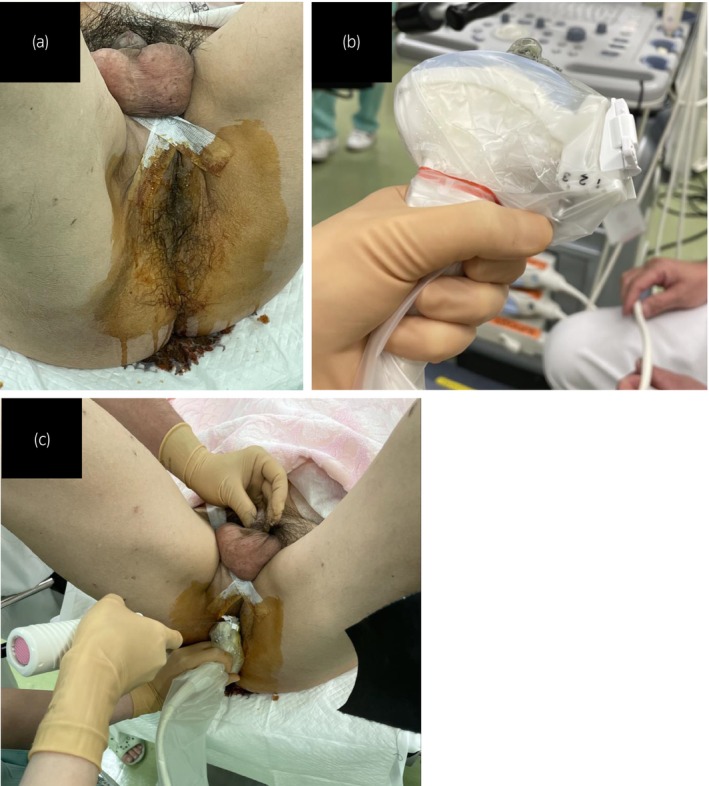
Transperineal approach for prostate biopsy. (a) The scrotum was retracted to expose the perineum. (b) TPUS scanning was performed using an abdominal ultrasound transducer with nephrostomy grid. (c) The biopsy gun was placed within the grid under ultrasound guidance, and prostate cores were obtained.
